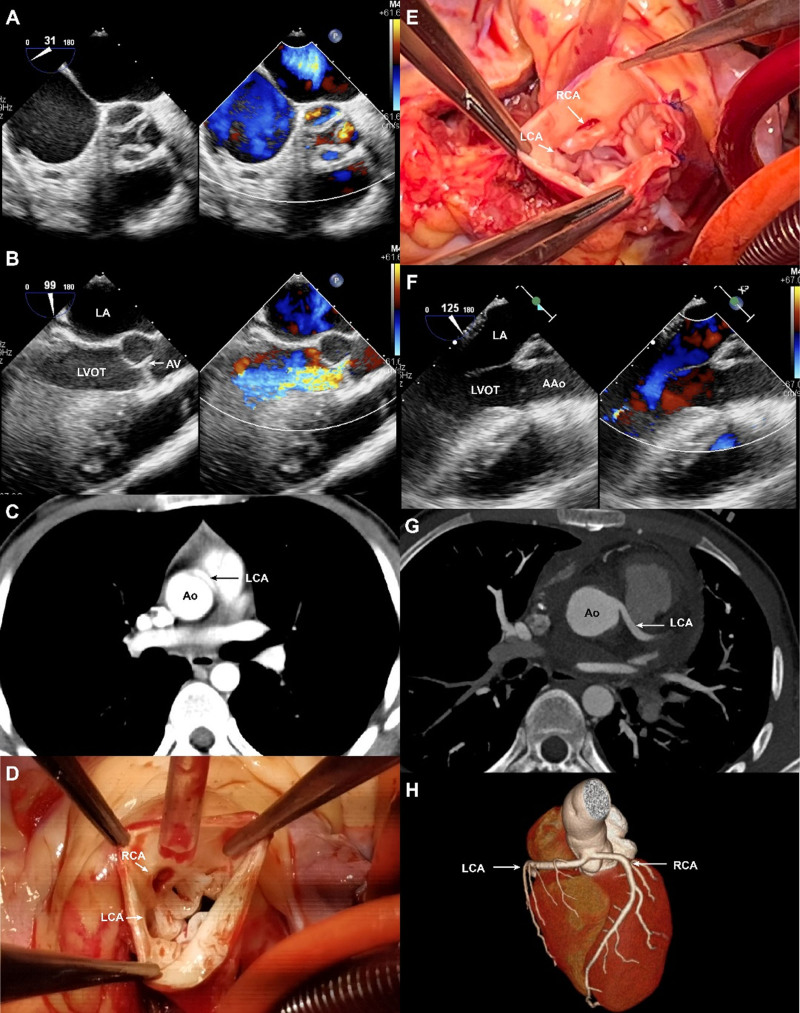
(A) A QAV in the short-axis view of the aortic root, (B) Aortic annular diameter was measured to be approximately 22 mm, (C) Cardiac-enhanced CT showed an anomalous aortic origin of the left coronary artery from the right coronary sinus with an intramural course, (D) The presence of QAV and double coronary artery ostia in the right sinus of Valsalva, (E) Pulmonary artery wall affixed below the coronary artery, (F) smooth LVOT, (G) and (H) Confluent ostium of coronary arteries and normal course of the coronary arteries. CT = computed tomography, LVOT = left ventricle outflow track, QAV = quadricuspid aortic valve.
